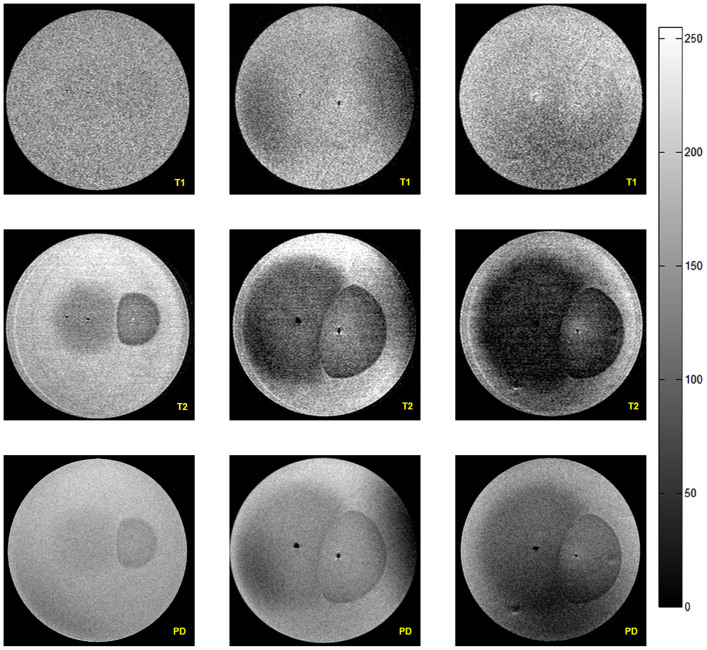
Comparative MRI imaging results. Each row corresponds to a sequence modality (Top to bottom: T1, T2, PD). Each column corresponds to a stimulation voltage (Left to right: 3 V, 6 V, 9 V).
